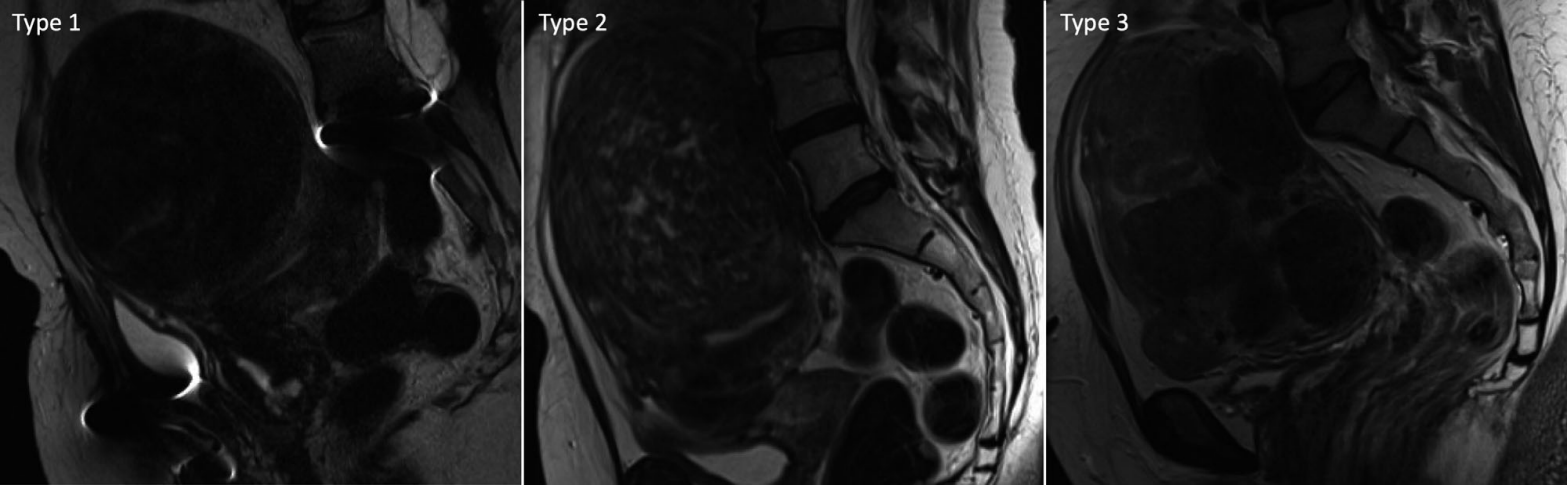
Possible examples of the Large Uterus Classification System preoperative appearance at the magnetic resonance imaging. Type 1: large uterus with voluminous anterior‐fundal fibroid. Anatomic insertions of adnexal vascular pedicles and uterine vessels are approximately at the same level as a normal‐size uterus. Type 2: large uterus with a voluminous antero‐fundal fibroid that cranially displaced the adnexal vascular pedicles insertion. Type 3: large uterus with multiple voluminous fibroids that cranially displaced the adnexal vascular pedicles insertion and distorted the pelvic anatomy with the displacement of uterine vessels.
